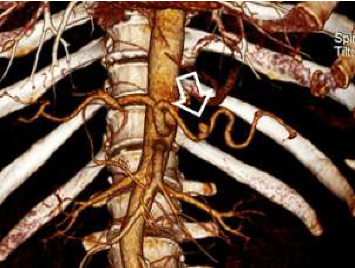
Multiplanar CT reconstruction demonstrating the pseudoaneurysm of the splenic artery (arrow).
